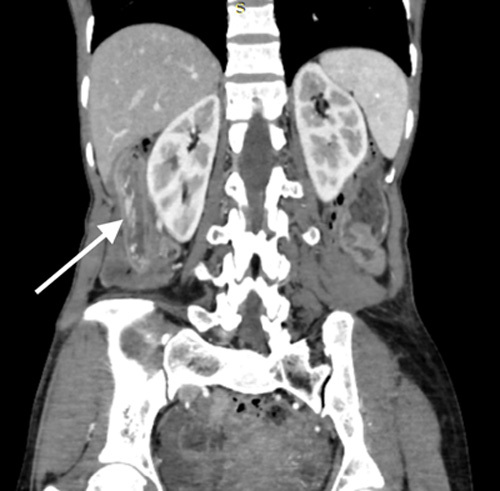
Contrast enhanced computed tomography (CECT) of the abdomen and pelvis in coronal reformation shows features of telescoping of distal ileum into caecum and ascending colon with an intussusceptum length of 13 cm (white arrow).
